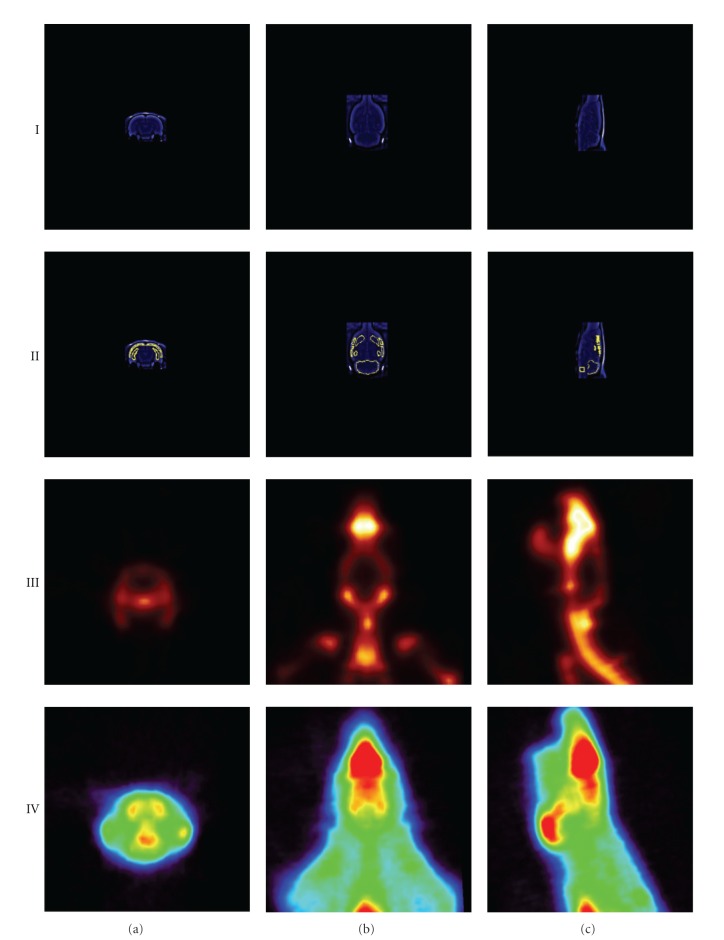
Overview of coregistered MR image and template with PET images. (I) MR image, (II) MR template, (III) [^18^F]NaF image, and (IV) [^11^C]AF150(S) image (0–45 min) for (a) coronal, (b) transverse, and (c) sagittal cross-sections.
